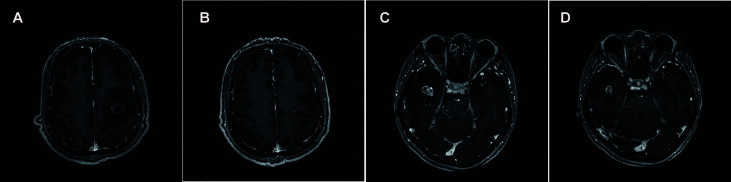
Brain MRIs of the patient revealed the size of BM lesion and PTBE. Comparing with the brain MRI before, the maximum vertical diameter of BM demonstrated slight shrunk (17×22 to 14×18 mm) (A, B) and (15×17 to 12×9 mm) (C, D) while peritumoral brain edema shrunk significantly.
